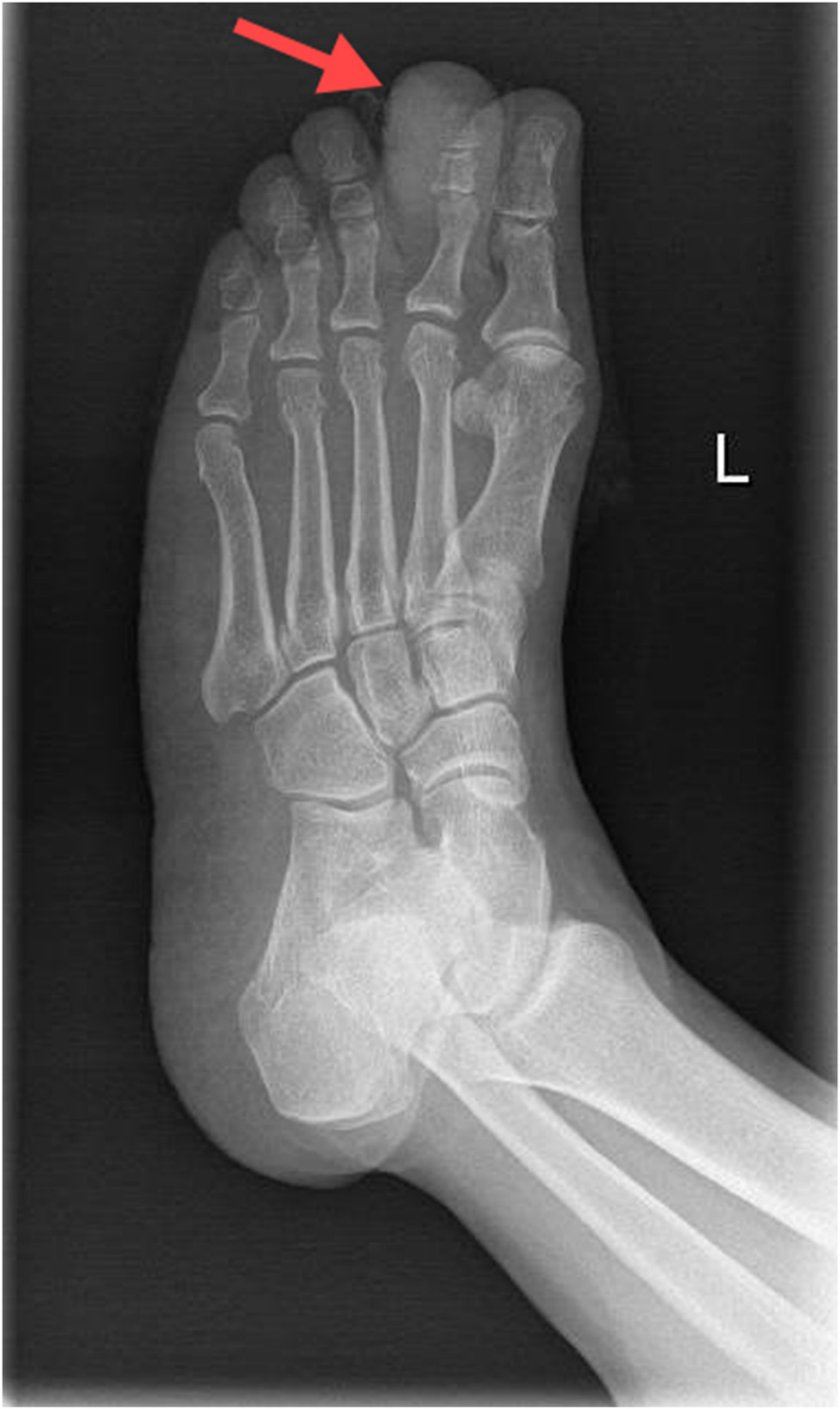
X-ray showed local bone destruction and soft tissue swelling in the distal phalanx of the second toe of the left foot in case 2.
